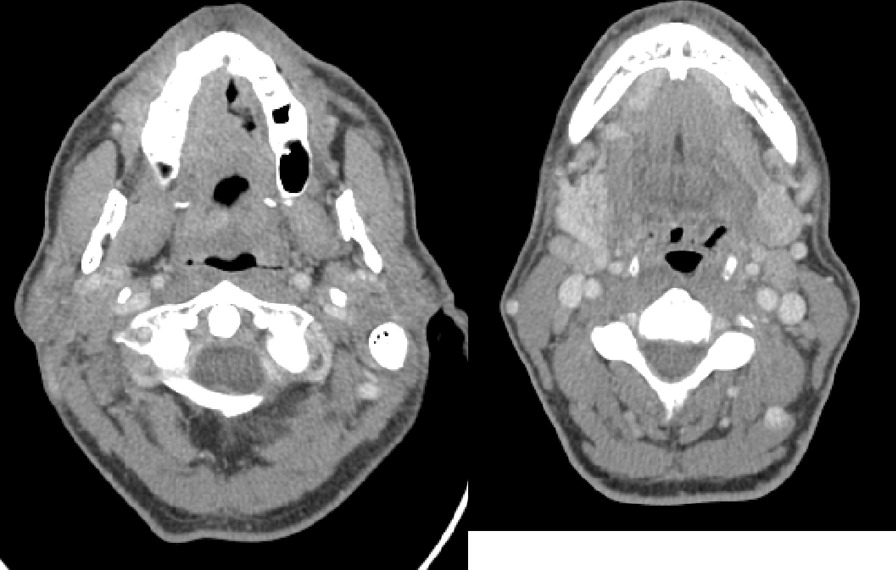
Post-radiation CT images with contrast
